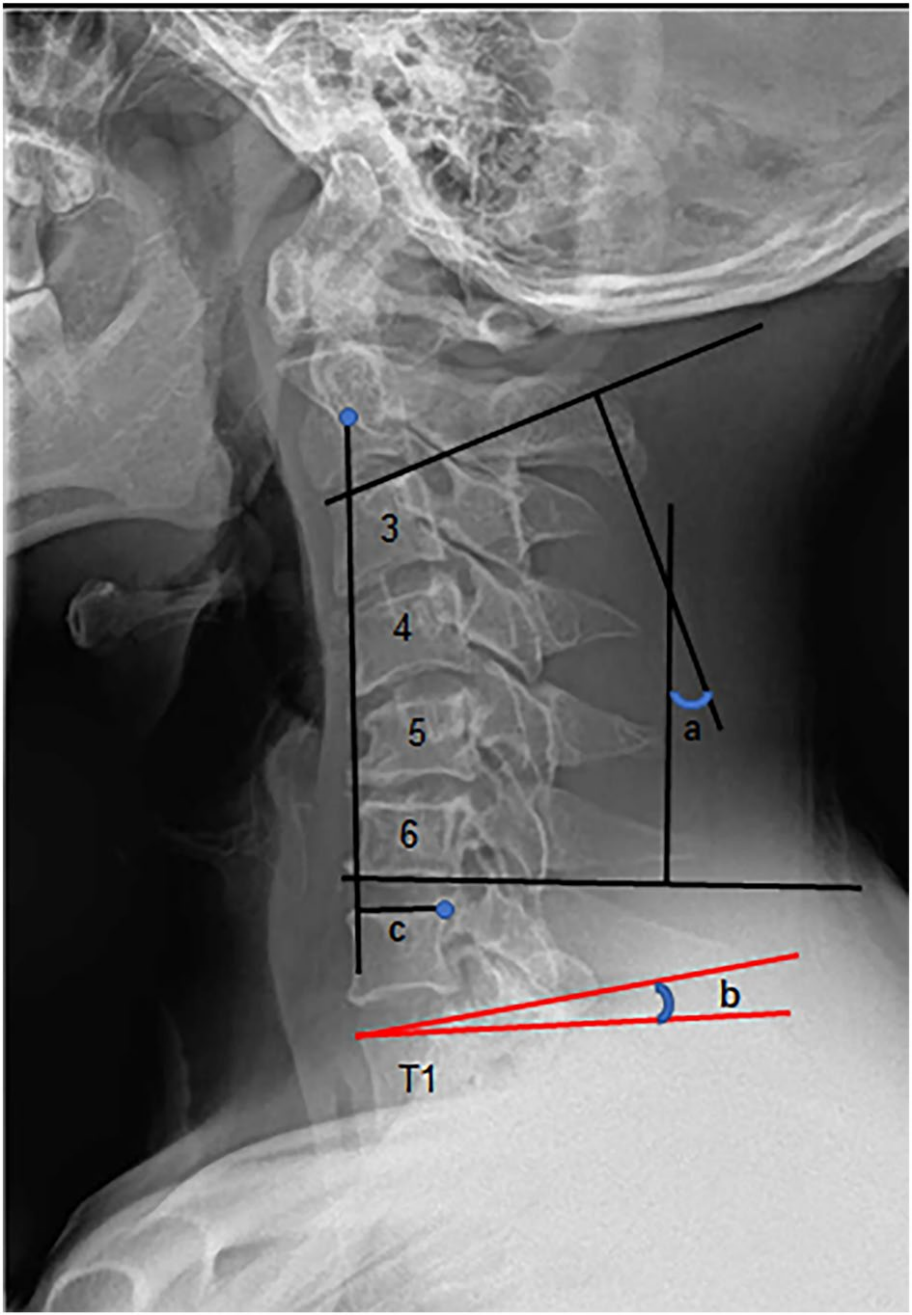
Radiographic measurements: (a) local Cobb angle (C3–6), (b) T1 slope, (c) C2–7 sagittal vertical axis.
